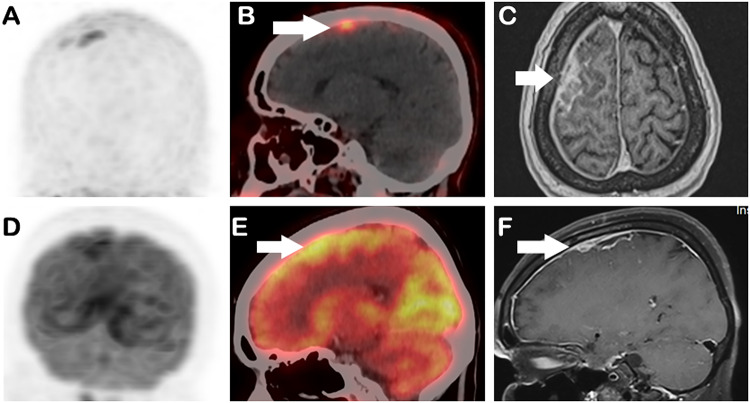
53-year-old female with Neuromelioidosis. MIP and sagittal fused ^68^Ga- FAPI PET/CT images shows focal tracer-avid meningeal enhancement in the right cerebral hemisphere along the right frontoparietal lobes with SUVmax 6.5 (A, B). Corresponding ^18^F-FDG PET/CT images shows mild FDG avid ill-defined enhancement in the same region with SUVmax 13.1, but not clearly distinct from the background uptake (D, E). MRI axial and sagittal post-contrast T1-weighted images shows diffuse pachymeningeal enhancement along the right cerebral hemisphere frontoparietal region confirming the disease involvement (arrows in C, F)
